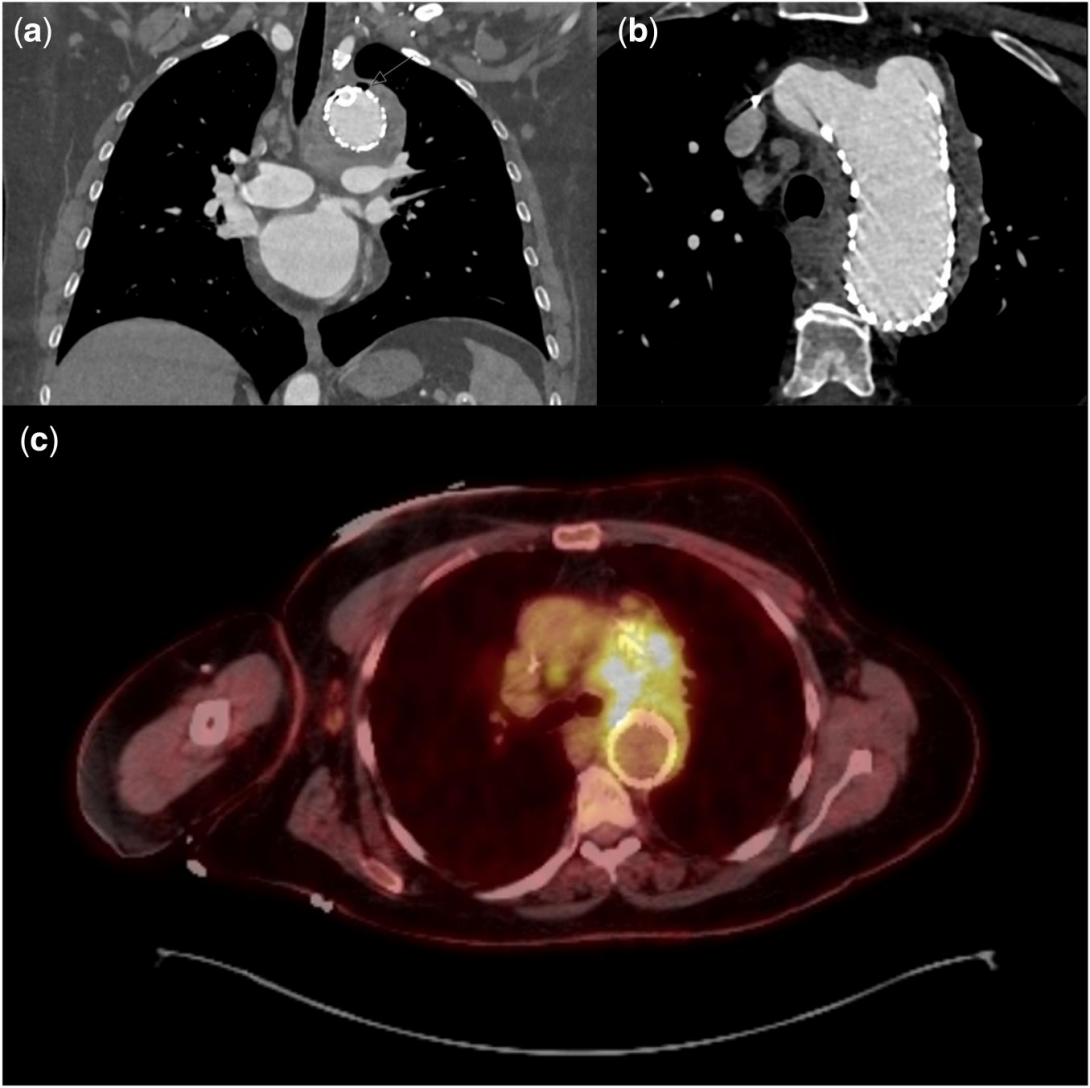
(a, b) CTA 2 months following TBE, demonstrating gas external to endograft with large pseudoaneurysm secondary to type 1a endoleak. (c) PET demonstrating significant FDG uptake at stented arch aneurysm. TBE: thoracic branched endograft
